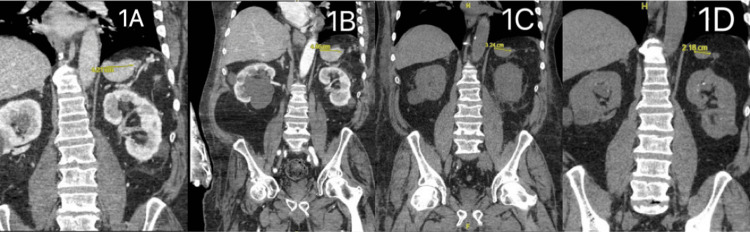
Computed tomography (CT) images display a potential accessory spleen located in the left upper quadrant of the abdomen (A) 08/23 - 4.21 cm, (B) 02/23 - 4.46 cm, (C) 2021 - 3.24 cm, and (D) 2016 - 2.18 cm.
